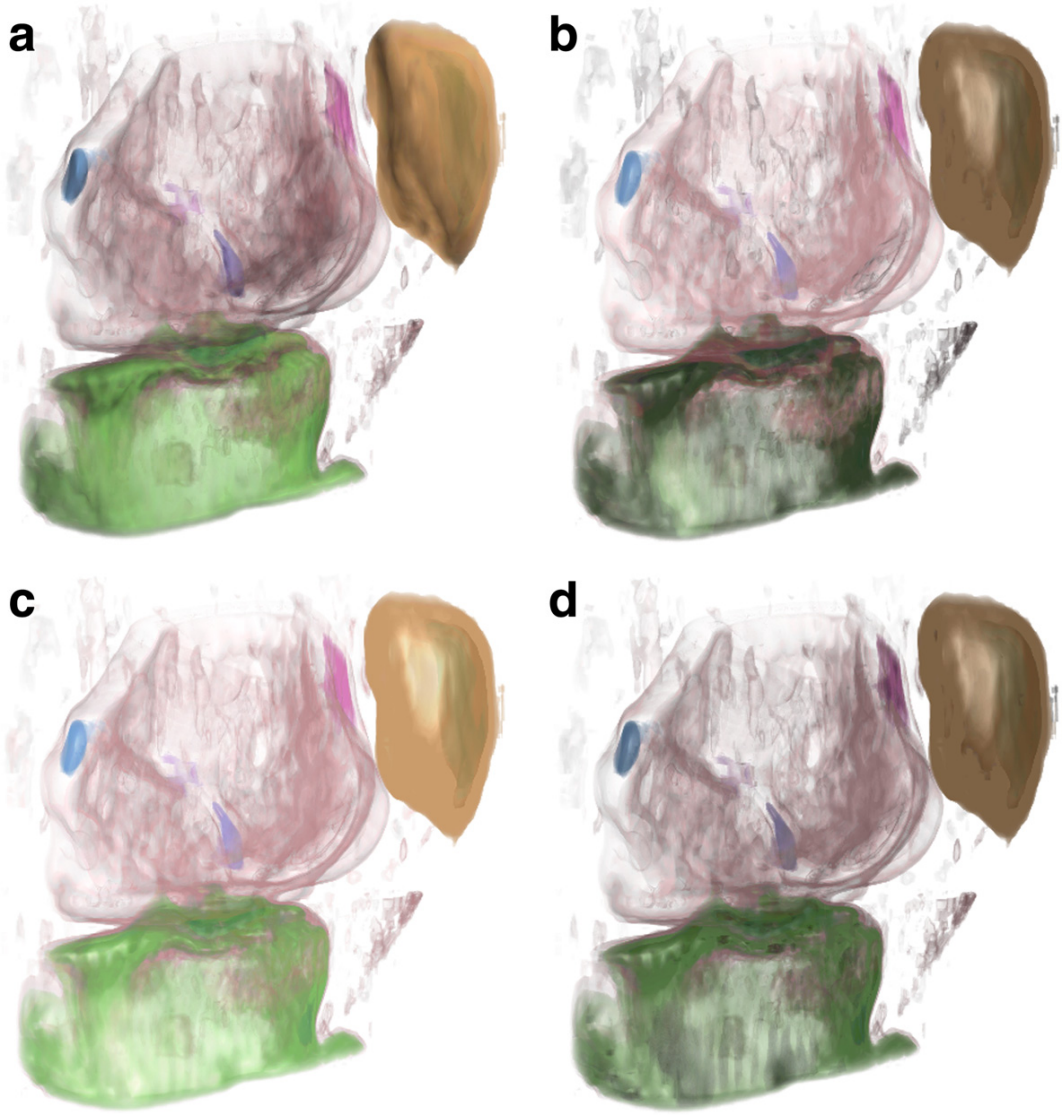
Volume rendering of CT-Knee data set with various illumination approaches: a Conventional illumination, b Importance illumination (persistence, *w* = 0.76), c Distance illumination (persistence, *w* = 0.76), d Perceptual illumination
